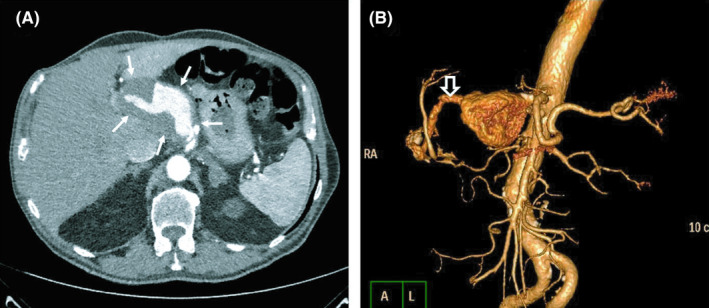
A, Axial and B, 3‐dimensional view of CTA showing a giant common hepatic artery aneurysm. The distal side of the common hepatic artery is shown (empty arrow)
